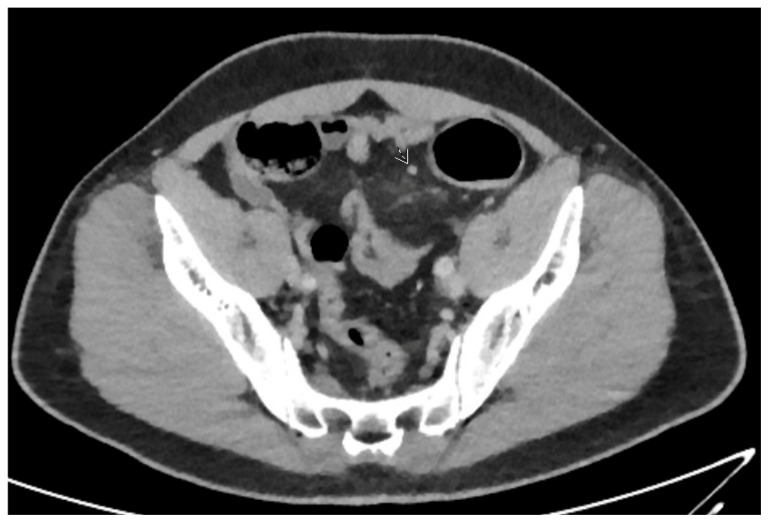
Axial contrast-enhanced CT of the pelvis demonstrating focal sigmoid colonic wall thickening with surrounding pericolic inflammatory stranding and a small adjacent gas-containing focus, compatible with acute diverticulitis.
